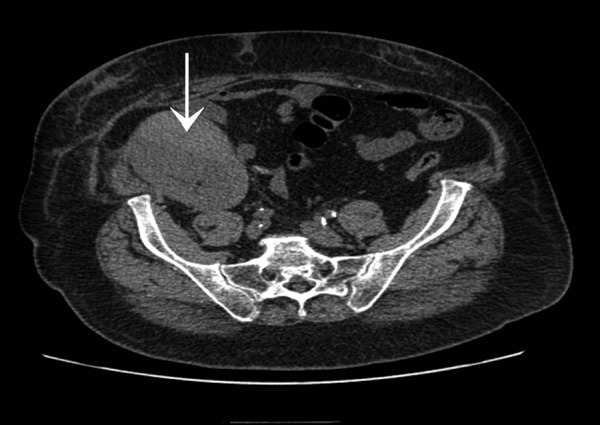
Plain CT scan showing subcapsular hematoma over anteromedial surface of transplanted kidney (arrow).
